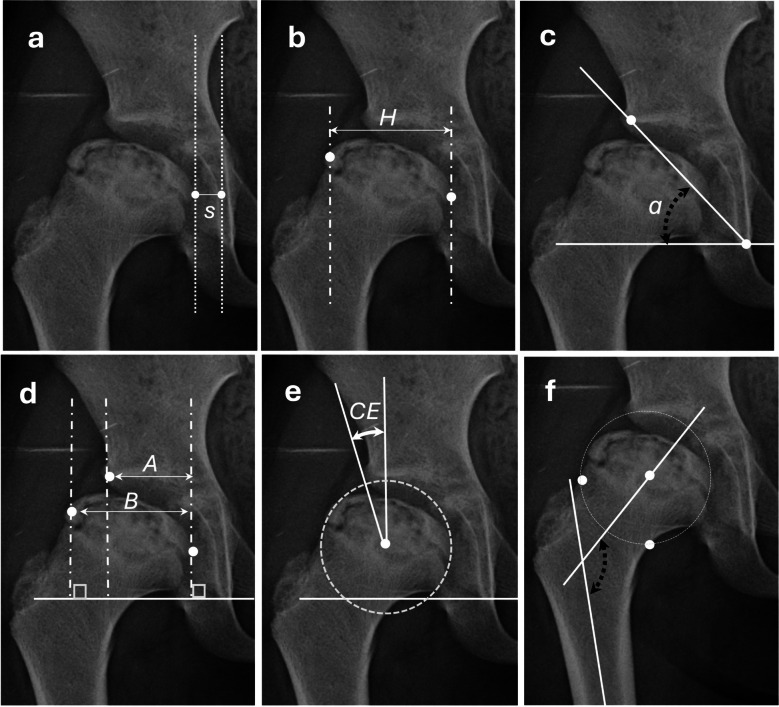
Representation of quantitative radiographic measurements (a) Femoral head subluxation ratio = S affectedside/S unaffectedside, b Femoral head size ratio = H affectedside/H unaffectedside, c Sharp angle (α), d Femoral head coverage ratio = A/B × 100, e Wiberg center–edge angle (CE), f Femoral neck-shaft angle (NSα)
